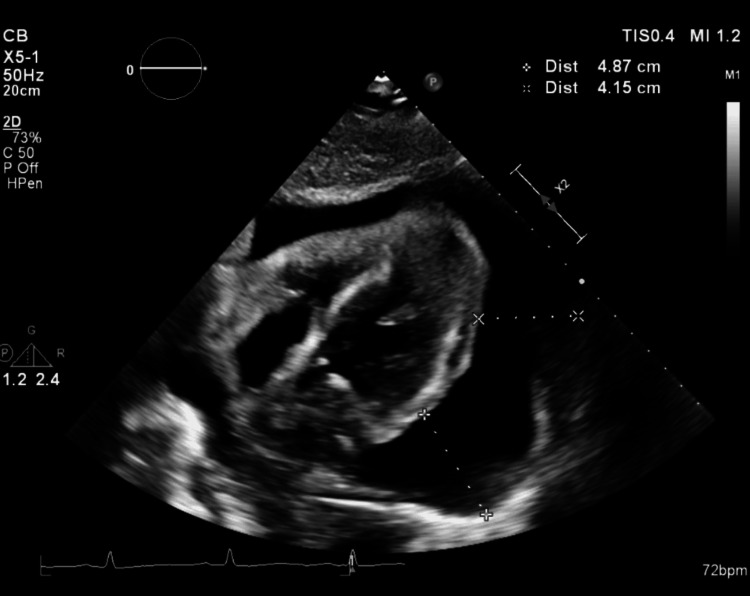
Transthoracic echocardiography demonstrates a large pericardial effusion with right atrial systolic collapse.
